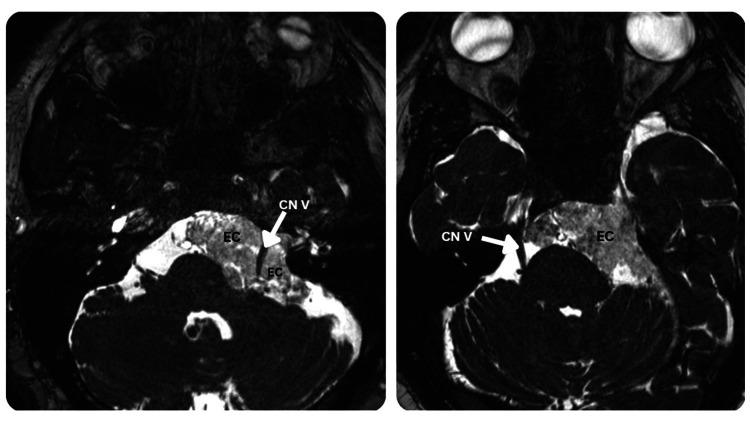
Axial T2-weighted MRI showing a cerebellopontine angle epidermoid cyst causing trigeminal nerve compression Axial T2-weighted images show a hyperintense lesion in the cerebellopontine cistern consistent with an EC. The lesion extends along the cerebellopontine angle and compresses the CN V at its root entry zone along the lateral surface of the pons. White arrows indicate the trigeminal nerve displaced by the epidermoid lesion. CN V, trigeminal nerve; EC, epidermoid cyst.
